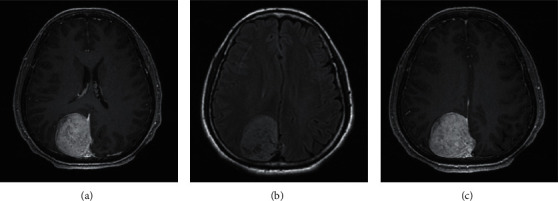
MRI image of transitional meningiomas (female, 28 years old, tumor on the right lateral ventricle). (a) Axial T2WI with low confounding signal. The tumor is elliptical, with clear borders, and no obvious peripheral edema. (b) Axial T1WI shows low signal, and the signal is uneven. (c) Axial T1WI shows obvious tumor enhancement.
